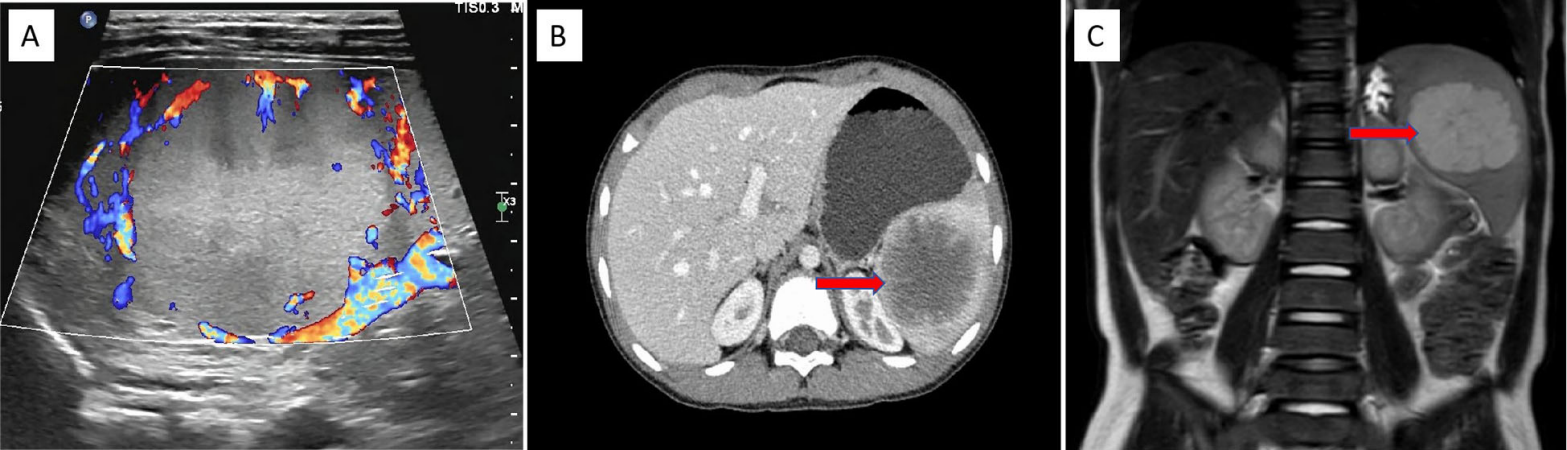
US, CT, and MRI findings of the splenic PILA. (A) Abdominal US image showing a medium echogenic area at the upper pole of the spleen, approximately 6.0 cm × 5.5 cm × 5.0 cm in size, with clear boundaries and abundant blood flow signals around the mass (coronal plane). (B) Abdominal CT image showing splenomegaly with a low-density mass visible within, approximately 6.3 cm × 5.7 cm × 5.2 cm in size, with relatively clear boundaries. After enhancement scanning, the edges and some internal parts of the lesion show mild to moderate enhancement (horizontal plane). This image displays a cross-section of the spleen. (C) Abdominal MRI image showing splenomegaly with clear boundaries, a slightly low signal in the center, and significant edge enhancement during the arterial phase of enhancement scanning. In the portal phase, there is progressive enhancement towards the center, resembling a spoke-like enhancement, with a signal higher than the splenic parenchyma. The central low signal shows no significant enhancement, and the lesion measures approximately 6.0 cm × 5.2 cm × 5.0 cm (coronal plane).
